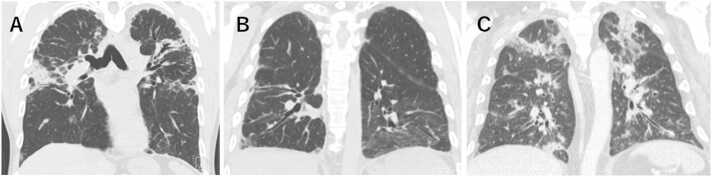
Distribution patterns of RAS-like opacities (RLOs) on CT in CLAD patients. (A) CT image demonstrating upper-predominant RLOs in a patient with a survival time of 5 years after CLAD diagnosis. (B) Lower-predominant RLOs with a survival time of 3 years after CLAD diagnosis. (C) Diffuse RLOs with a survival time of 5 months after CLAD diagnosis. CLAD, chronic lung allograft dysfunction; CT, computed tomography; RAS, restrictive allograft syndrome; RLOs, RAS-like opacities.
